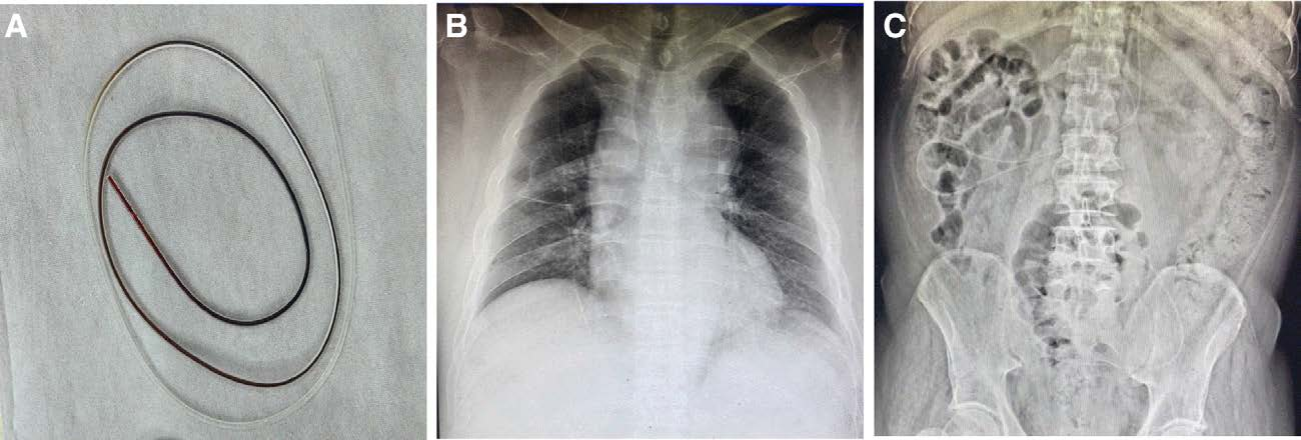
(A) After removal of the mediastinal luminal shunt, a thrombus was visible inside the shunt. (B and C) Postoperative chest and abdominal radiographs showing proper positioning of the abdominal end of the shunt bilaterally. CT = computed tomography.
